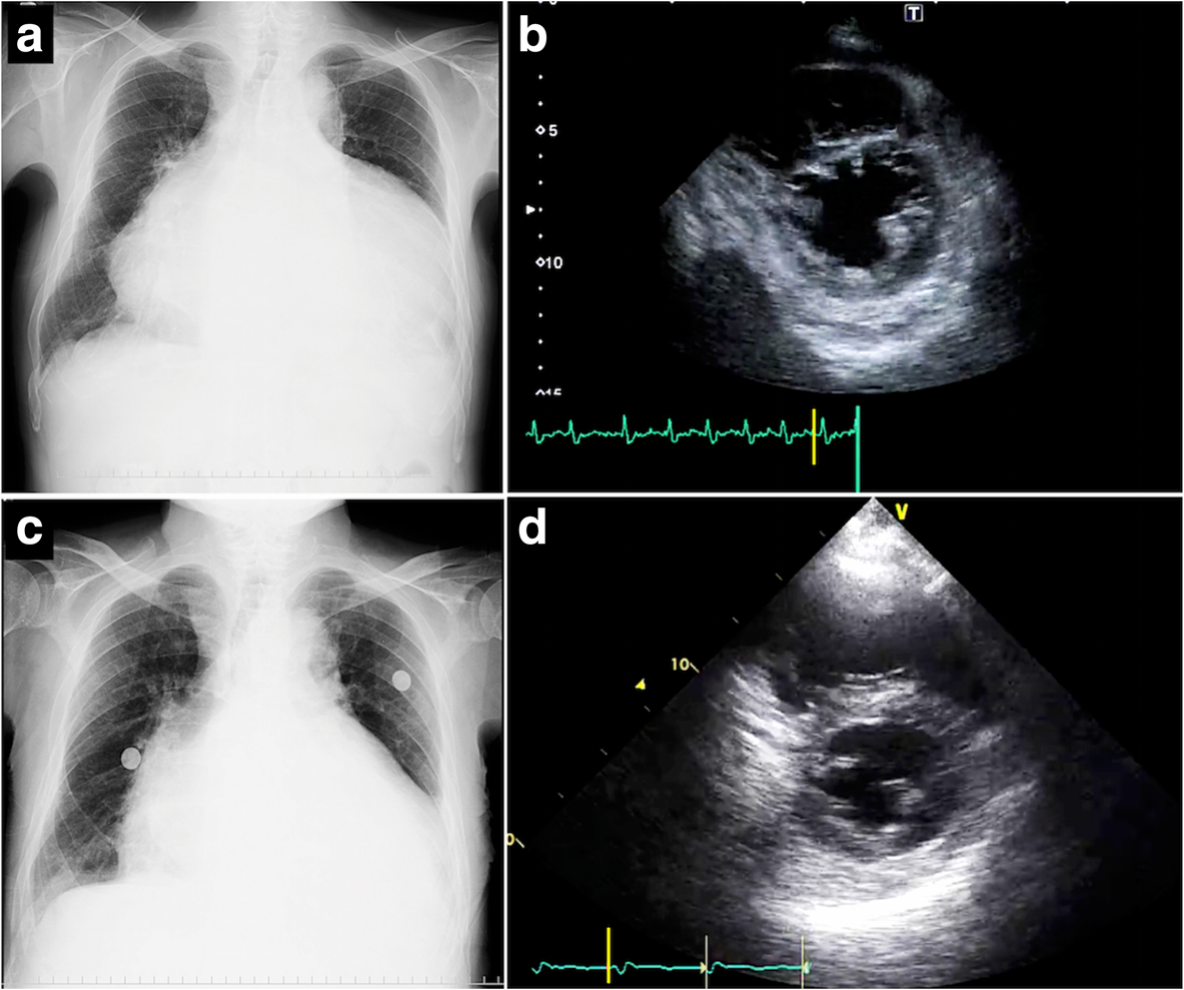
The findings of chest X-ray and transthoracic echocardiography during hospitalization. a The chest X-ray on the first day of hospitalization showed an increased cardiothoracic ratio of 84.4% and bilateral pleural effusion. b The end-diastolic ventricular septal shift was still present after removal of the pericardial effusion, as evaluated by transthoracic echocardiography. c A chest X-ray after the administration of oral corticosteroid therapy detected a reduced cardiothoracic ratio of 73.4%. d Transthoracic echocardiography after the administration of oral corticosteroid therapy detected that the diastolic ventricular septal shift was improved at discharge
